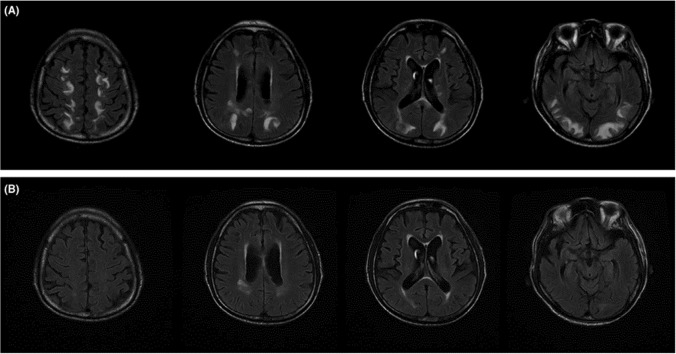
ICIs-related posterior reversible encephalopathy syndrome. A T2 FLAIR (fluid‐attenuated inversion recovery)‐weighted magnetic resonance imaging showing subcortical and deep white matter high signal intensity involving both cerebral hemispheres, especially temporooccipital and high frontoparietal areas. B Two‐week follow‐up study showing decreased extent of high signal intensity lesions in subcortical and deep white matter of both cerebral hemispheres (with permission from Kim D. et al. “Posterior reversible encephalopathy syndrome induced by nivolumab immunotherapy for non–small-cell lung cancer”. [38])
